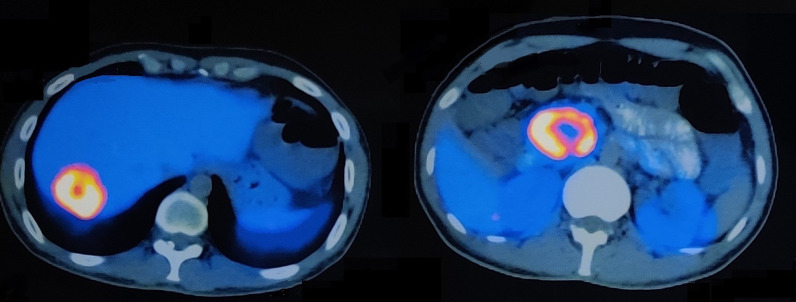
Axial section images of ^68^Ga DOTA-NOC PET CT scan showing enhancing lesions in the segment VII of liver (SUV max-14.7) and head of pancreas (SUV max-38.2)
